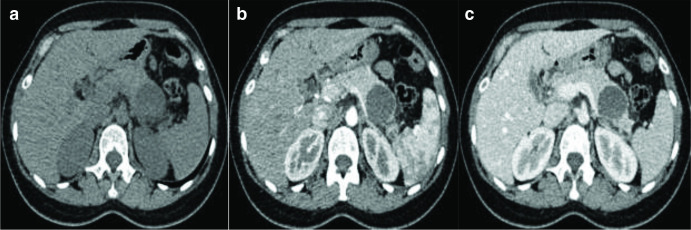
A. Axial non-enhanced CT section of the upper abdomen showing a cystic lesion
